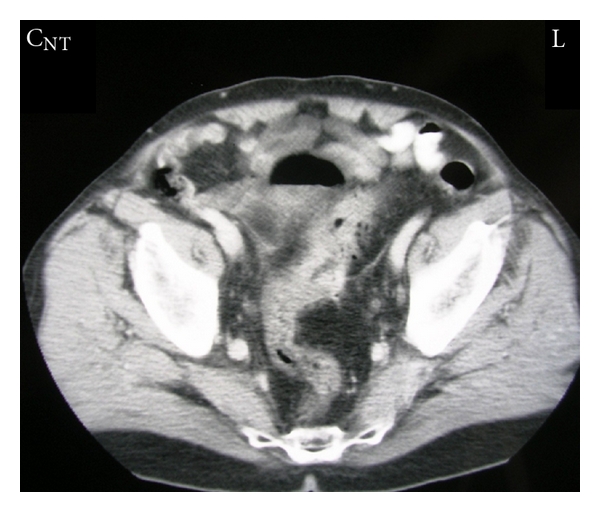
Transverse abdominal CT scan demonstrating airlevel into the urinary bladder closely in contact with a thickened segment of the sigmoid colon and colon diverticula.
